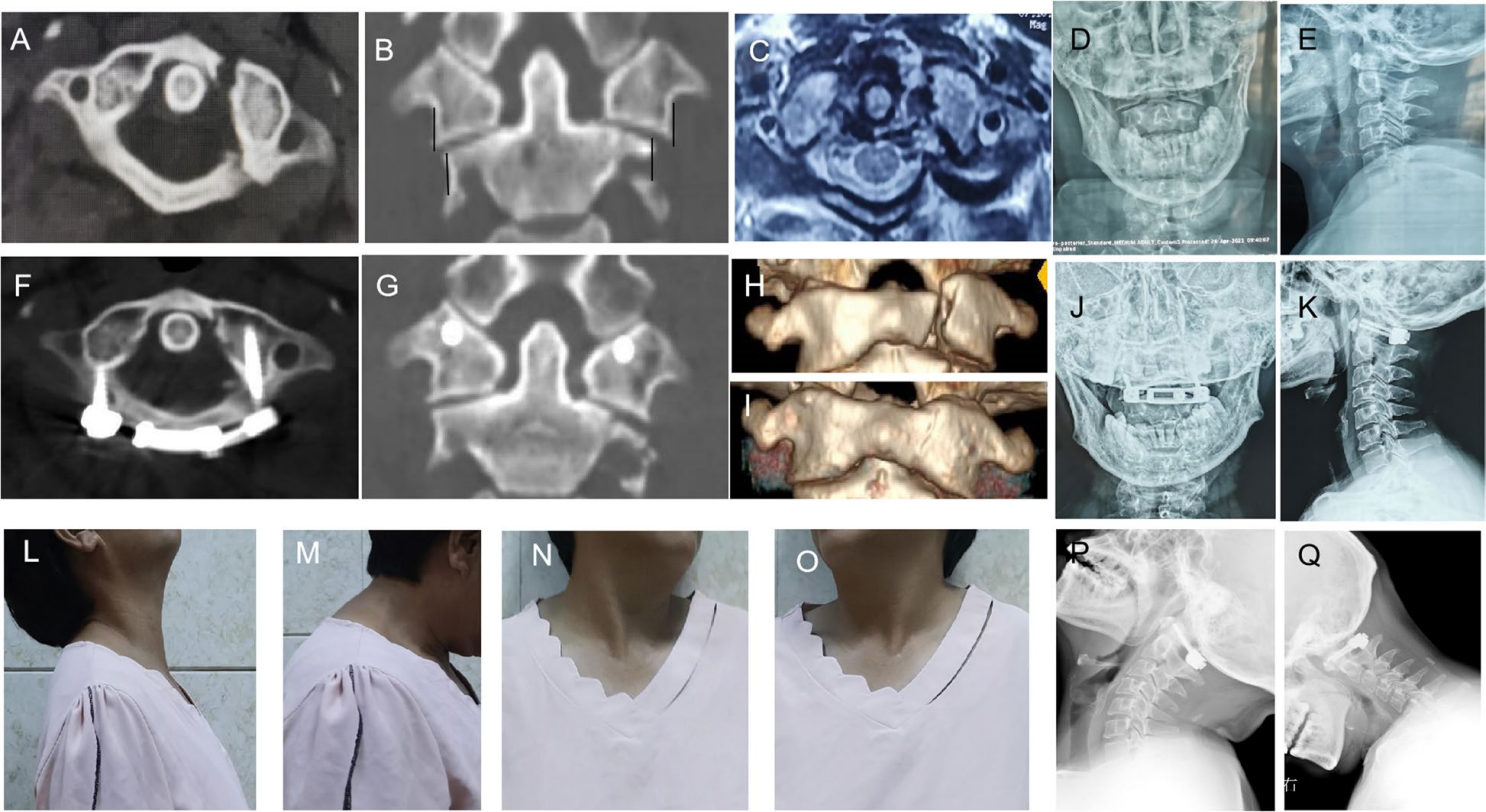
A 50-year-old woman with C1 left anterior and posterior arch fractures (A, H), lateral mass displacement (B, D, E) and Type II TAL injury (C). Postoperative CT scans and 3-D reconstructed images revealed satisfactory reduction (F, G, I).The lateral and open-mouth X-ray image (J, K) at 2 months after surgery revealed that the screws of the atlas lateral masses were placed sufficiently, and the fixation system was not loose. The patient completely recovered the full range of motion of the cervical spine without pain at the last follow-up (L-O). The lateral radiograph of the fexion-extension cervical spine demonstrated good atlantoaxial stability without dislocation (P-Q)
